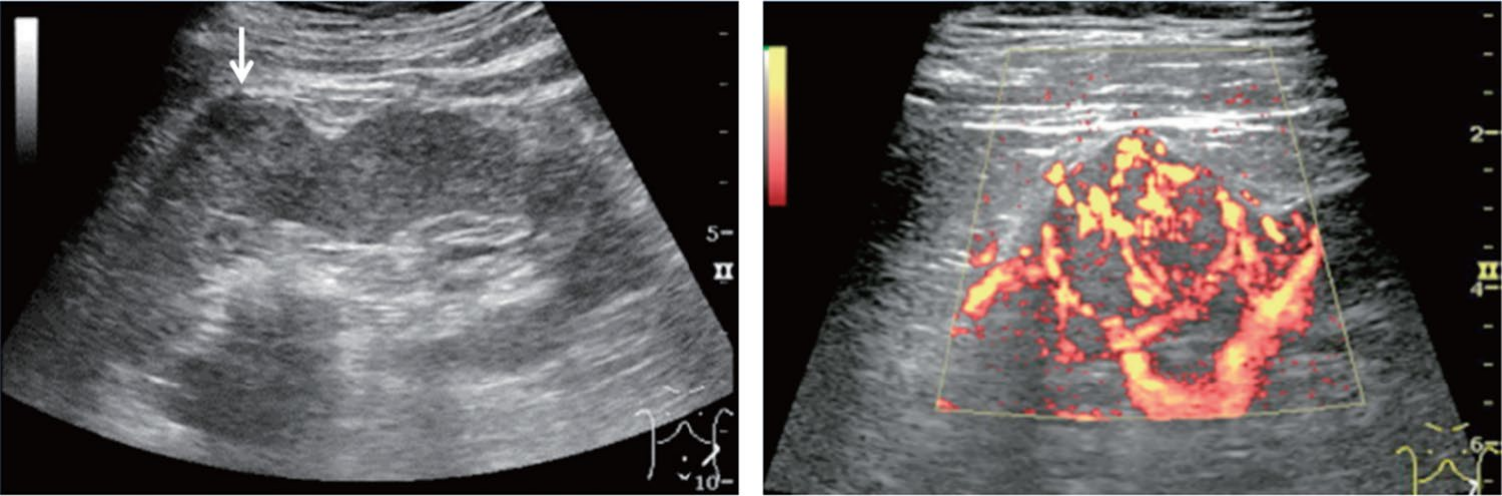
Morphological abnormality. Rugged contour is present (basket-like blood flow is displayed in lesion on color Doppler). Left: convex probe; right: power Doppler with high-frequency probe. Category 3, Assessment D2
